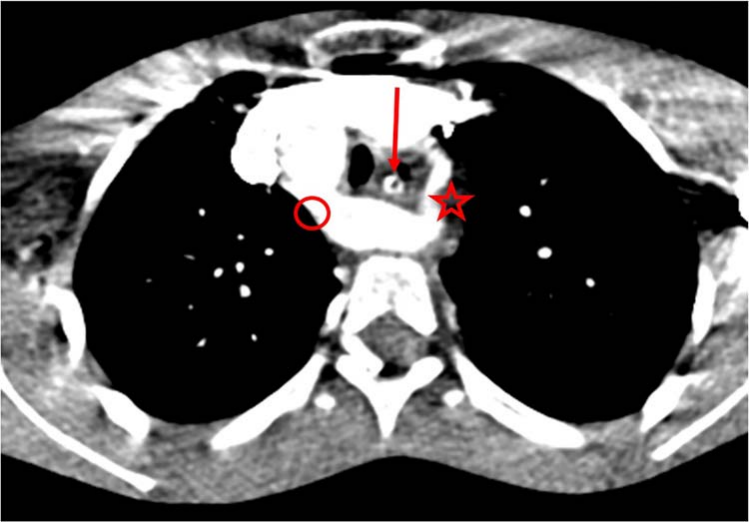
Double aortic arch on thoracic angio-CT scan: arrow (esophagus), star (left arch), circle (right arch).
